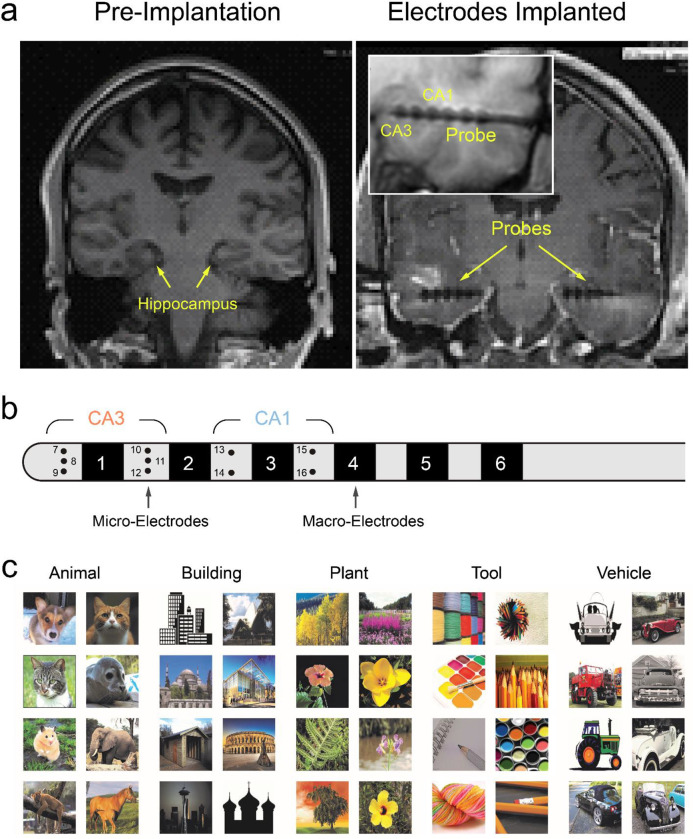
Human experimental paradigm. a: 3T MRI showing pre-implantation hippocampal structures and post-implantation electrode locations in one subject. Inset: zoomed-in view of the probe in the hippocampus. b: Layout of the micro-macro probe containing 6 macro-electrodes and 10 micro-electrodes. Six and four micro-electrodes were implanted in the CA3 and CA1 regions, respectively. c: Sample Images of the five memory categories used in the DMS task.
